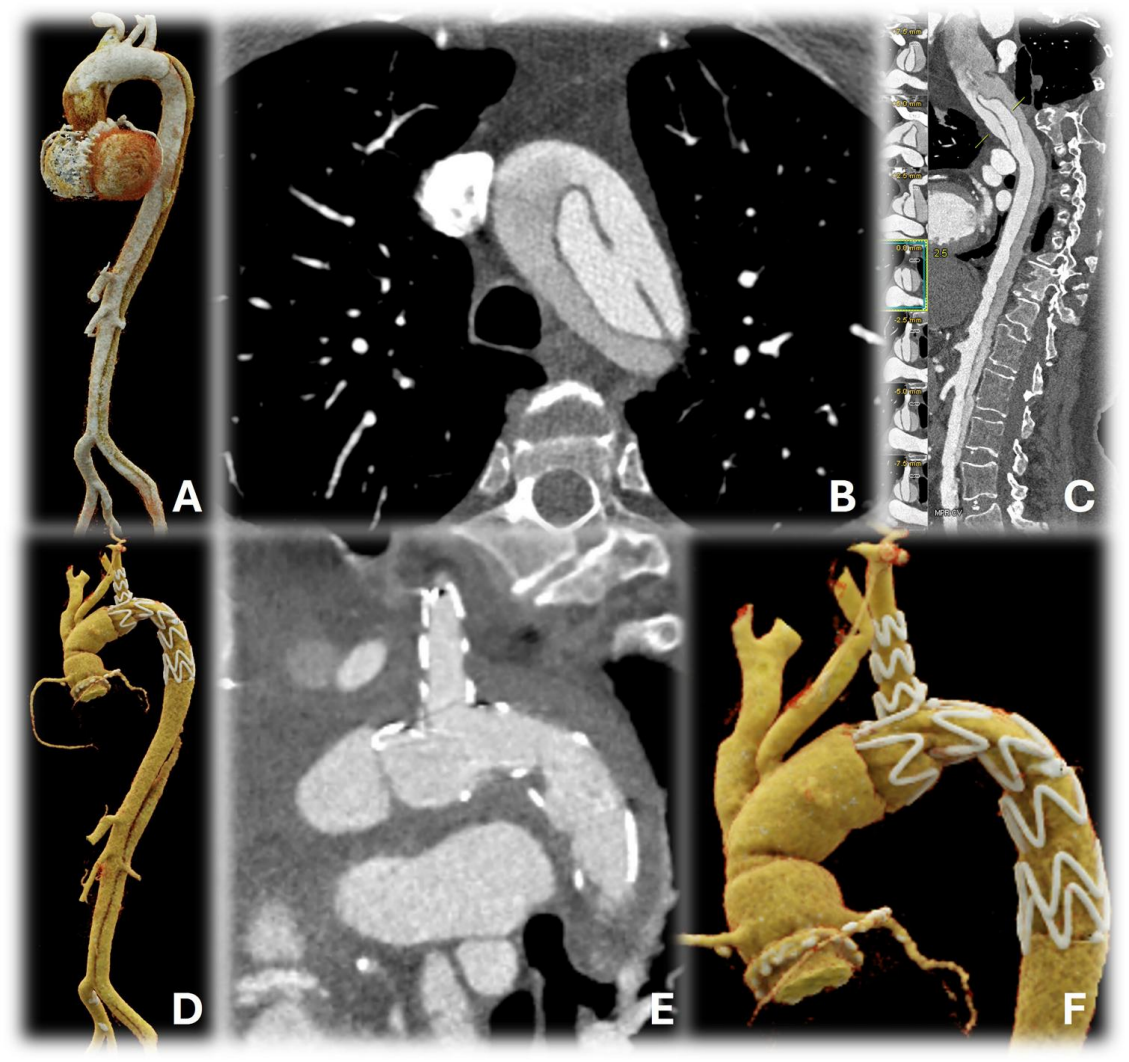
Pre- and Postoperative Computed Tomography Angiography. Preoperative 3D reconstruction of the aorta. Primary entry tear in the distal aortic arch. Centerline reconstruction. Postoperative 3D reconstruction of the aorta. (B) Complete stent unfolding. (C) Satisfactory remodeling and no signs of endoleak
